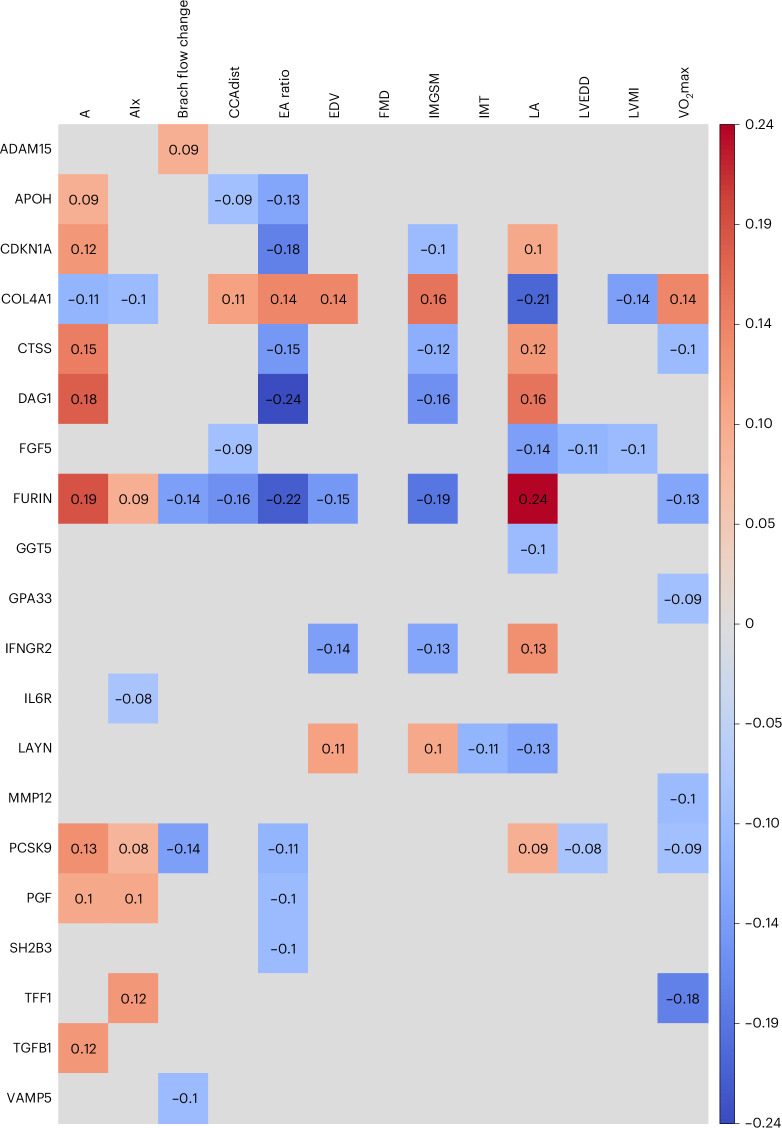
Heat map showing cross-sectional relationships between plasma levels of 20 proteins of interest and subclinical markers of CVDs. Multivariable linear regression was used and the regression coefficients are shown for relationships with *P* < 0.05 (two sided). A gray square indicates *P* ≥ 0.05. A, transmitral A-wave at echocardiography; AIx, augmentation index at radial artery pulse wave analysis; Brach flow change, the increase in brachial artery blood flow following 5 min of blood flow arrest; CCAdist, carotid artery distensibility by ultrasound; EA ratio, left ventricular diastolic function index; EDV, acetylcholine-mediated increase in forearm blood flow; FMD, brachial artery FMD; IMGSM, echolucency of the carotid artery intima–media complex; VO_2_max, maximal oxygen consumption at an exercise test. For complete protein names, see Supplementary Table 16. Source data
